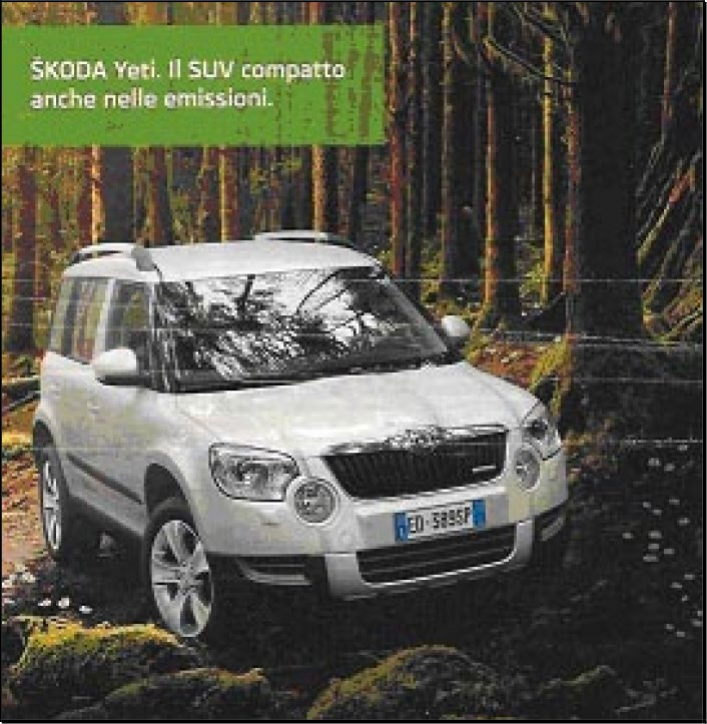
“Škoda Yeti. Il SUV compatto anche nelle emissioni.” (The SUV which is compact also in emissions)
